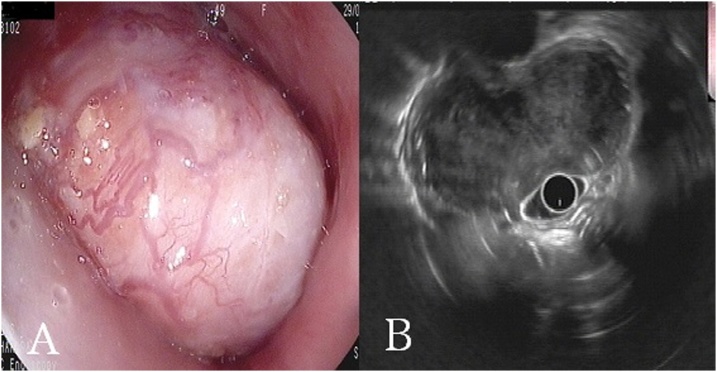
Upper gastrointestinal tract endoscopy revealing a protruding submucosal lesion at the proximal segment of the esophagus with an intact and hypervascular mucosa (A). The soft tissue mass is almost obstructing the esophageal lumen. (B) An endoscopic ultrasound image of the well-defined mass almost concealing the esophageal lumen.
